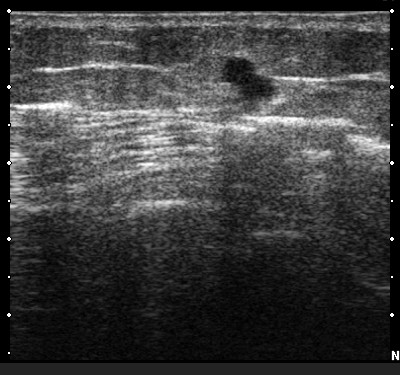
Intraoperative ultrasound showing an 8 mm hypoechoic lesion identified within the subcutaneous tissues of an area of generally increase ^18^F-FDG activity within the anterior lower one-third of the left thigh region.
